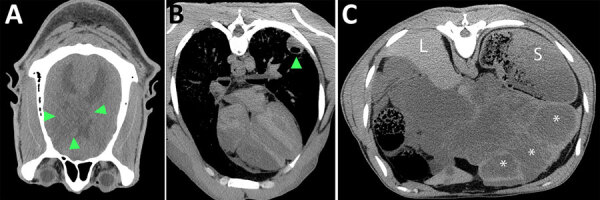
Postmortem computed tomography images in soft tissue window from a case of neurologic alveolar echinococcosis in postpartum zoo-housed gorilla, the Netherlands, 2024. A) Transverse view of the brain (anterior is bottom, right is left of image). Green arrows show space-occupying lesion within right hemisphere of cerebrum. B) Transverse view of the lung shows fluid- and gas-filled lesion within the left lung lobe (arrow). C) Transverse view of the liver shows multiple thick-walled, fluid-filled lesions (asterisks). L indicates normal liver tissue; S indicates stomach.
